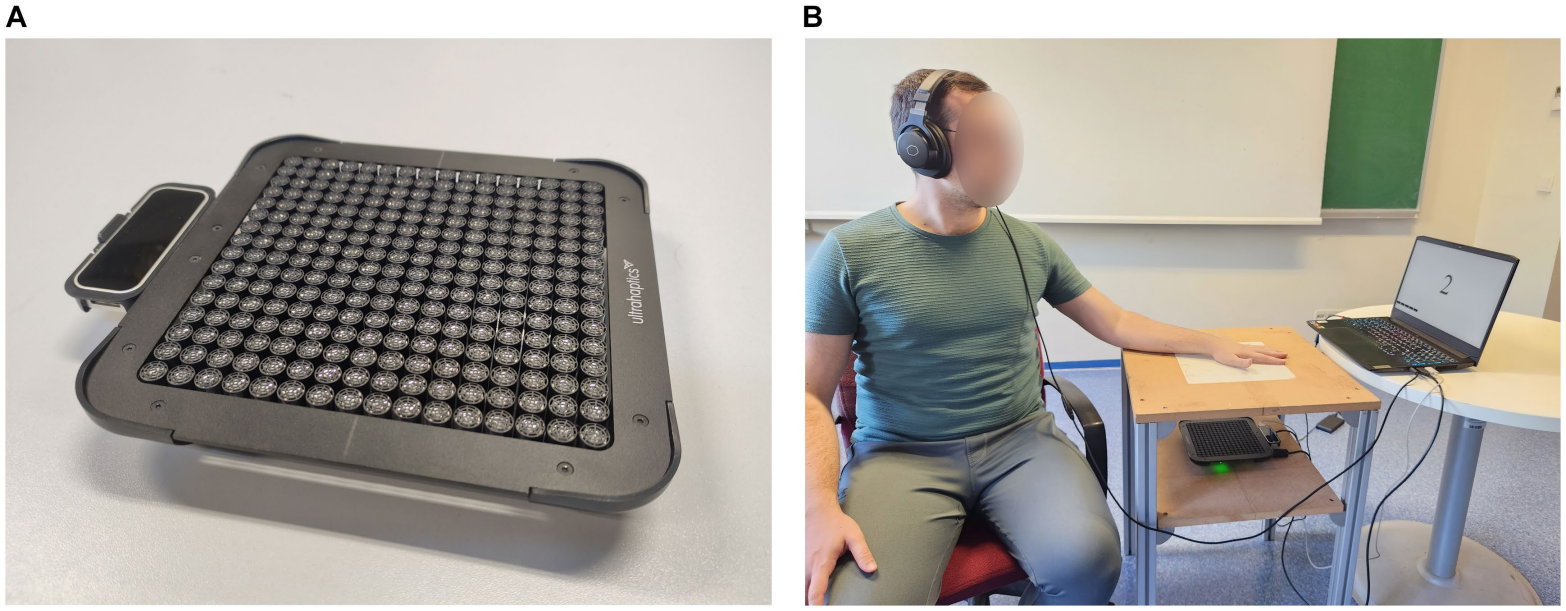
Mid-air haptics device and the experimental setup. (A) The mid-air haptics device (Ultrahaptics STRATOS Explore) consists of an array of ultrasound emitters. Also attached to the system is a hand tracking device (Leap Motion Controller) with infrared light emitters and cameras. (B) The device was placed on a stand with an adjustable distance to hand platform. The hand platform included a Plexiglas cover with different diameter holes for exposing the stimulus location on the skin to amplitude modulated ultrasonic waves. The device was controlled by specialized software on a personal computer. During the psychophysical experiments, the participants sat in a comfortable chair and wore headphones with white noise to mask auditory cues from the device. The task intervals were indicated on the computer screen. The participant responses were acquired verbally and entered to the computer by the experimenter.
